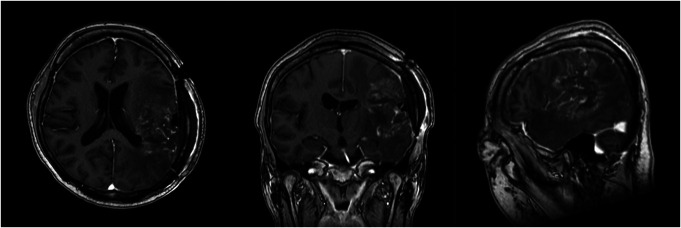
Axial, coronal, and sagittal gadolinium-enhanced T1-weighted images taken 1 day after the second surgery.
